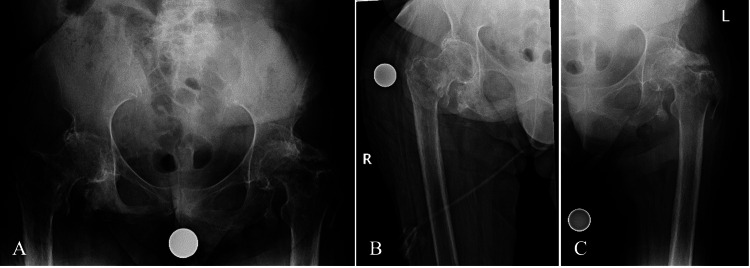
Initial injury radiographs A) Anteroposterior view of the pelvis demonstrating a left intertrochanteric (AO: 31A2.2) and right intertrochanteric (AO: 31A2.1) femur fracture. Anteroposterior view of B) the right hip and C) the left hip further demonstrates the fracture patterns. AO: Arbeitsgemeinschaft für Osteosynthesefragen
